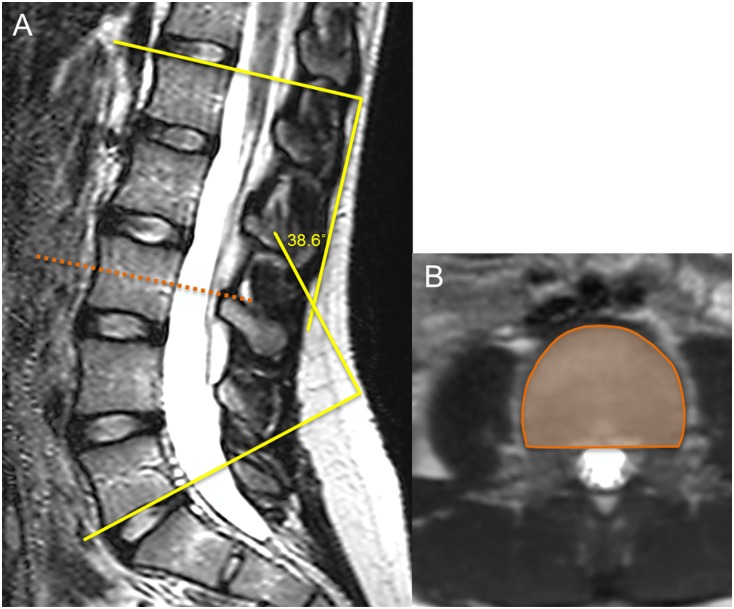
MRI images of the lumbar spine in a 13-year-old girl. (A) Coned-down sagittal image of the lumbar spine showing the degree of lumbar lordosis measured as the angle between the superior endplate of L1 and the inferior endplate of L5. (B) Axial image outlining the measurement of vertebral CSA at the third lumbar vertebra.
